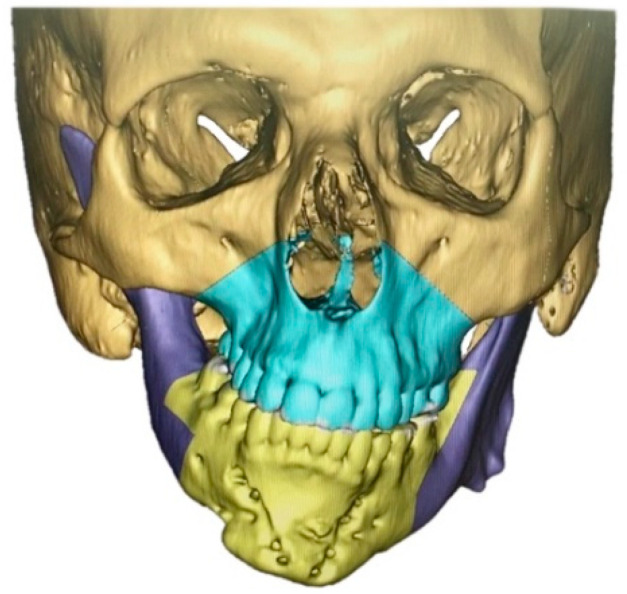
Preoperative virtual planning. Baseline situation as identified by CBCT. Color-coded: maxilla (turquoise), corpus mandibulae (yellow), and rami mandibulae (purple).
